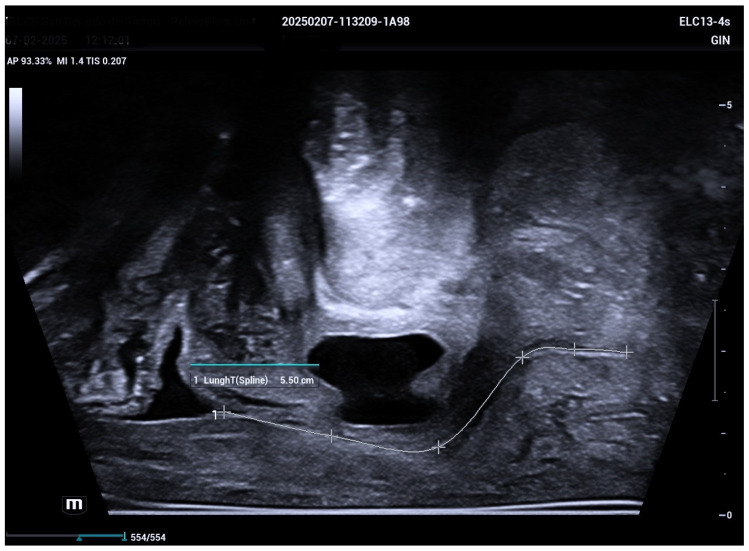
Mid-sagittal imaging using a biplanar ultrasound probe demonstrates a urethral diverticulum located approximately 1.5 cm distal to the internal urethral orifice. The diverticulum measures 3 cm in length and causes a marked 90-degree angulation of the distal urethra. The total length of the urethra is measured at 5.5 cm. This anatomical distortion may contribute to urinary symptoms and complicate vaginal delivery.
